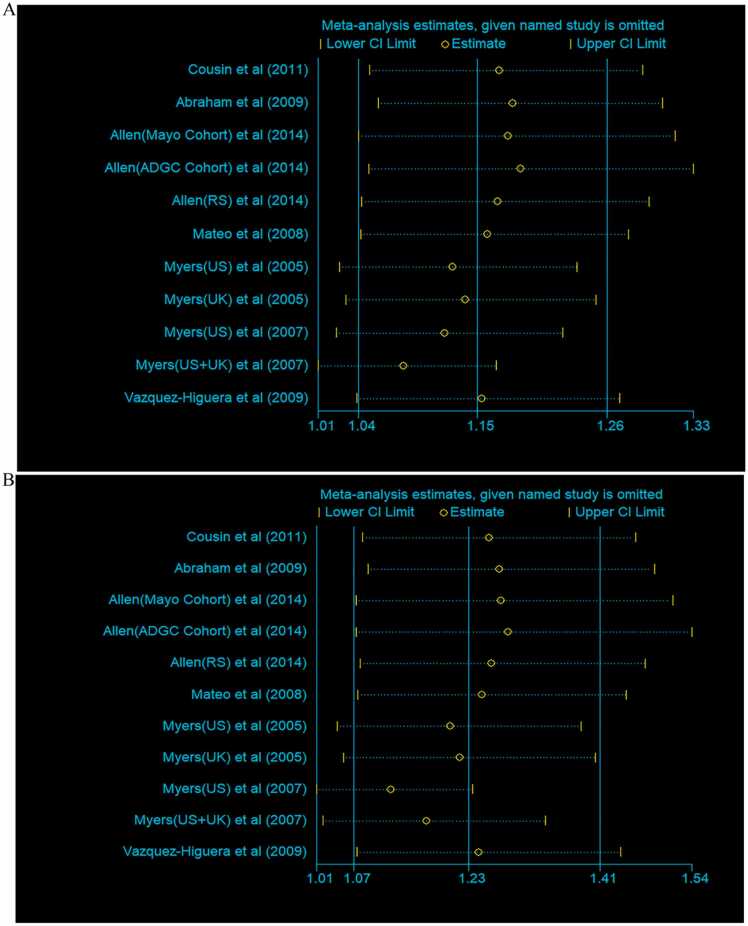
Sensitivity analysis of studies on the association between rs2471738 and Alzheimer's disease.(A) Based on the Tvs.C model. (B) Based on the TT+CT vs.CC model.
